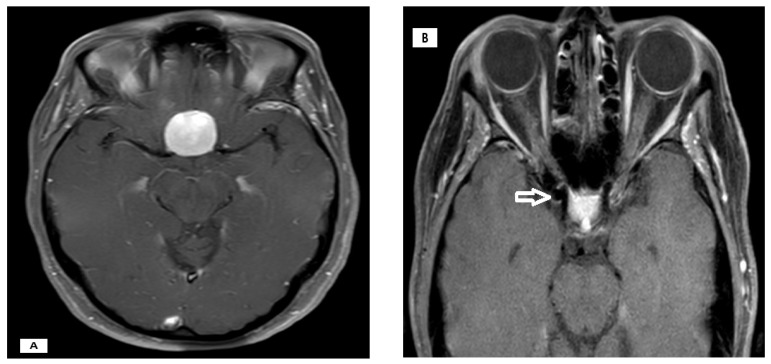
A 41-year-old female patient underwent cranial MRI as part of the diagnostic evaluation for visual disturbances. Imaging demonstrated a meningioma of the tuberculum sellae (A) and incidentally revealed a paraclinoid aneurysm of the internal carotid artery (as indicated by arrow) (B). The meningioma was resected, and in the same surgery, the aneurysm was treated by microsurgical clipping.
